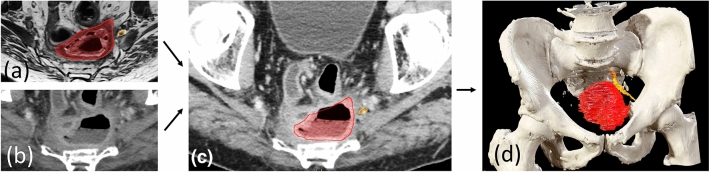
Preoperative segmentation and virtual 3-dimensional (3D) model. a Tumour (*red*) and the adjacent S2 nerve (*yellow*) manually segmented on axial MRI. b Axial CT image. c Segmented structures transposed to axial CT image after fusion of MRI and CT. d Virtual 3D model with automatically segmented pelvic bone and manually segmented tumour and S2 nerve
